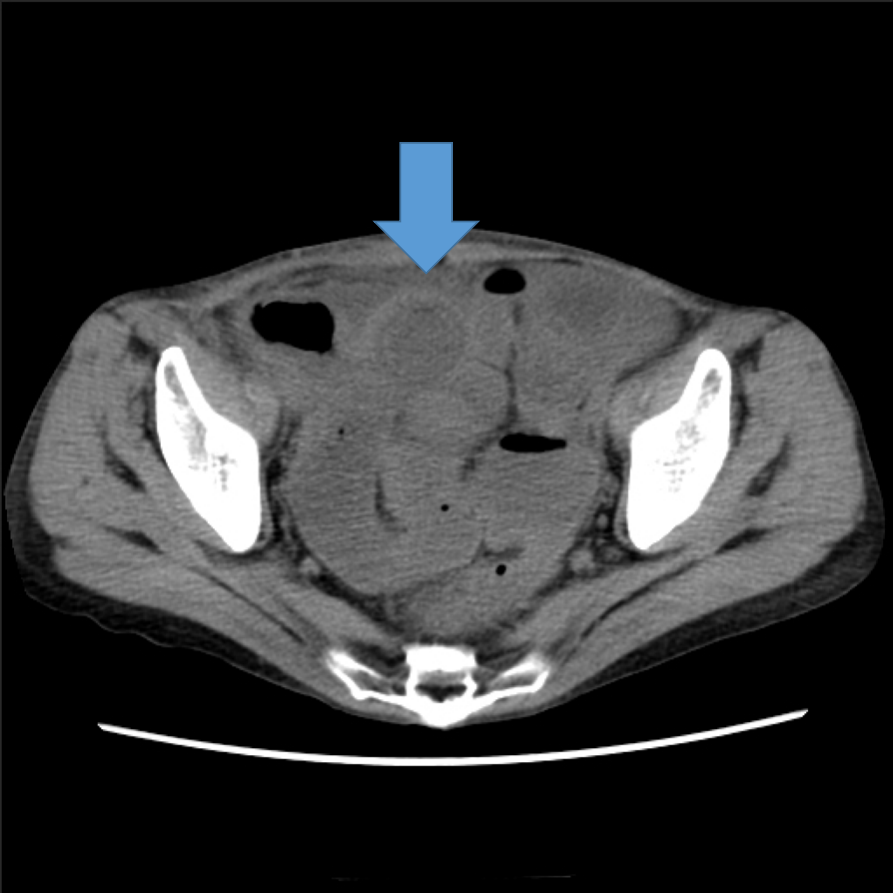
Abdominal computed tomography scan. Intestinal fluids are accumulated in the small intestine, and the target sign can be observed (arrow)
